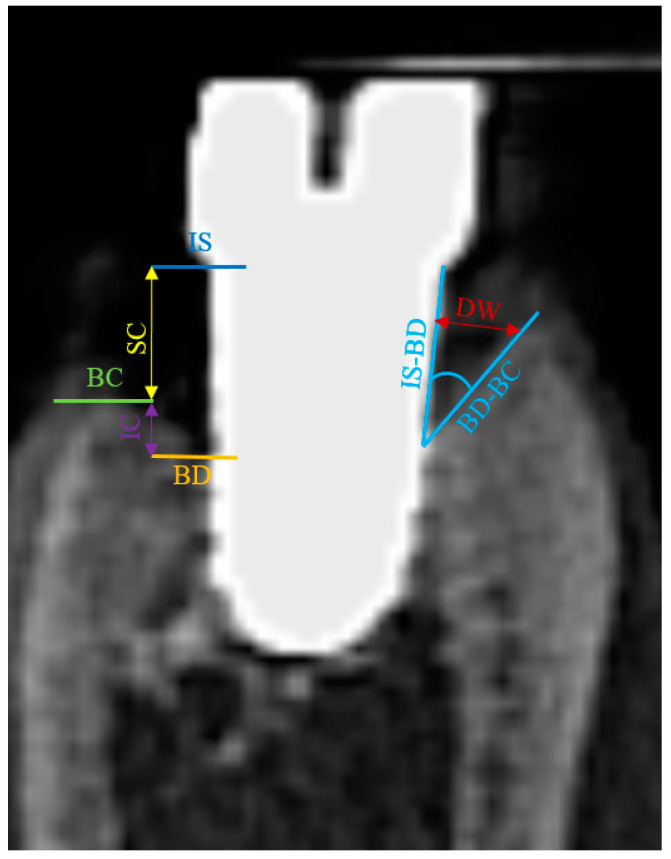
Landmarks identified in each sagittal image at all buccal, oral, mesial and distal aspects: IS (implant shoulder), the bottom of the bone defect (BD), the alveolar bone crest (BC) and the angle between segments IS-BD and BD-BC. CBCT implant #5 (original magnification ×8).
